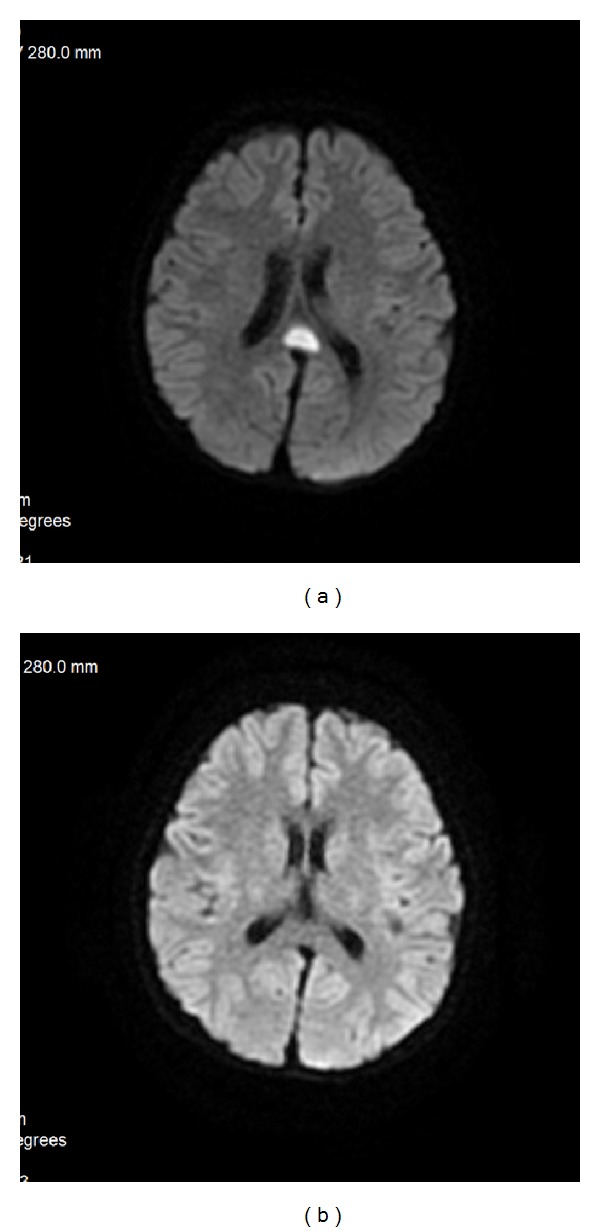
DWI sequence in axial plane. (a) Transient splenial lesion in a 9-year-old boy with school problems visualized as a hyperintense focus in the midline. (b) Six months later the lesion is absent.
